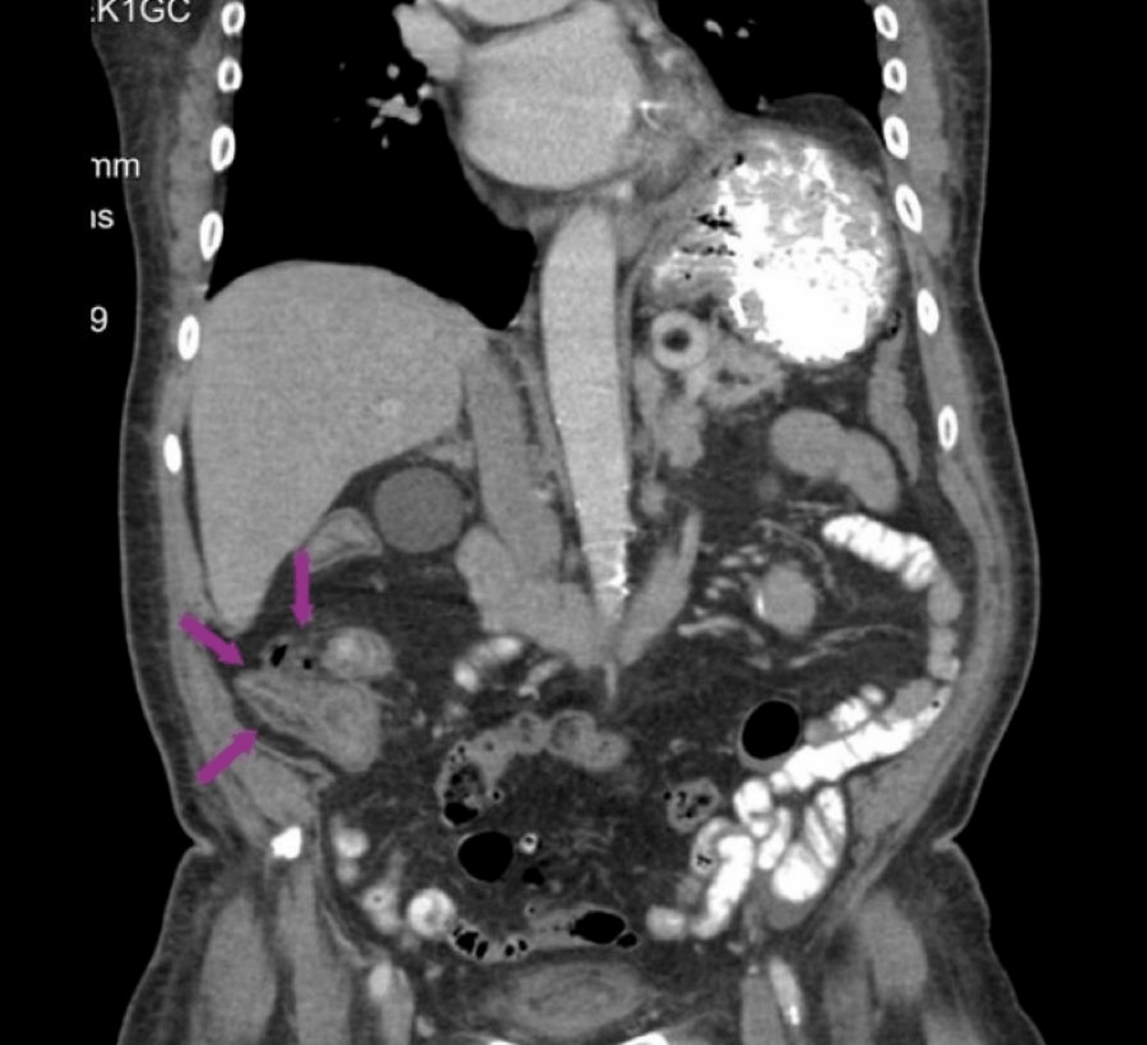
Contrast-enhanced CT of abdomen and pelvis (coronal view) two months prior to current presentation, displaying abscess (purple arrows) of the appendix
